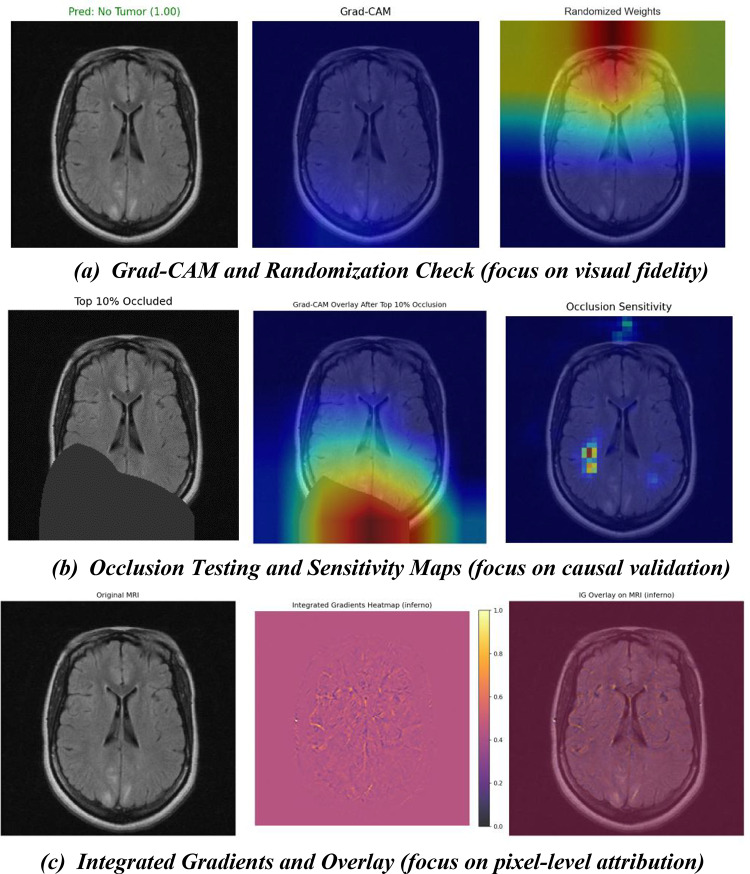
Representative Example of XAI on Brain MRI Non-Tumor Case from Internal Test Dataset (a) Grad-CAM highlights tumor regions, while randomization disrupts activations. (b) Occlusion testing and sensitivity maps confirm causal relevance of highlighted areas. (c) Integrated Gradients overlays, visualized with a perceptually uniform colormap (Inferno), provide pixel-level attribution consistent with Grad-CAM, reinforcing interpretability reliability.
